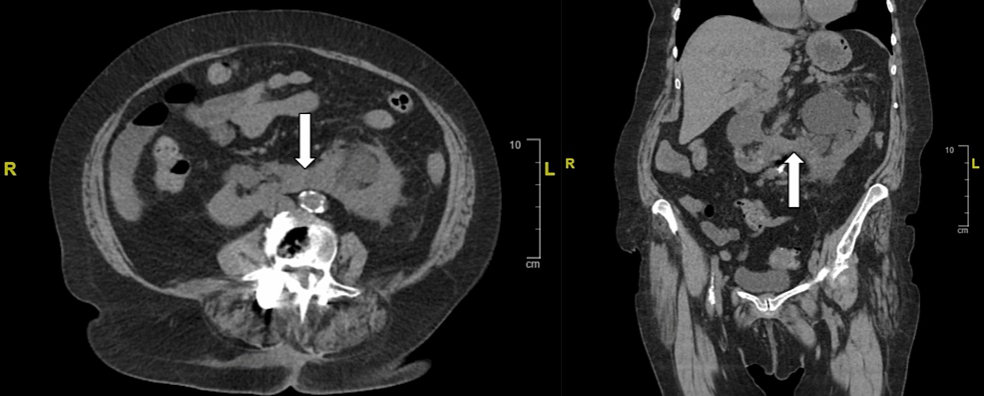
CT abdomen/pelvis without contrast in axial (left) and coronal (right) views showed an HSK (white arrows). HSK: Horseshoe kidney
